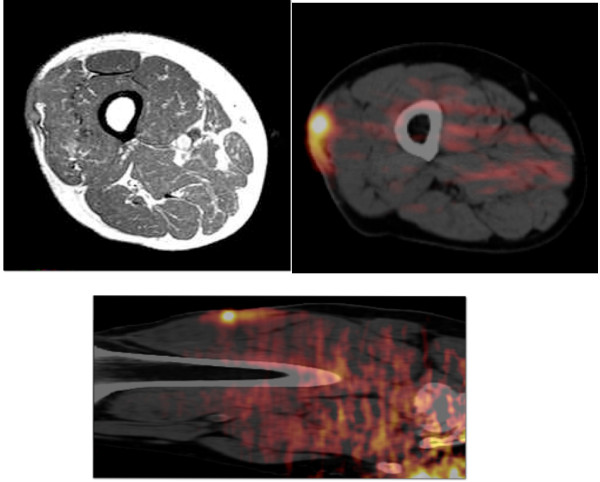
MRI & PET-CT. A: MRI image demonstrates a small recurrent tumor on the vertical surgical margin at the fascia lata of the right thigh. B: PET-CT mages in coronary (1) and sagittal (2) sections of the right thigh and hot spots indicate the recurrent tumor.
